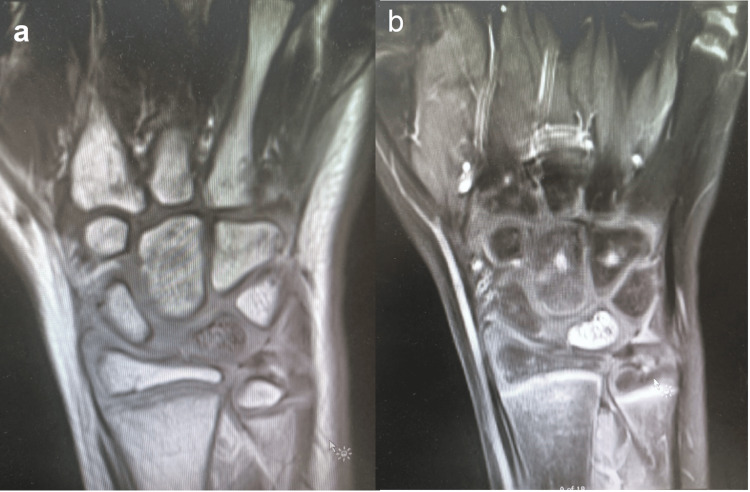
The altered bone marrow signal of lunate bone, which appears hypointense on T1-weighted image (a) and hyperintense on short tau inversion recovery (STIR) (b), represents a bone marrow edema but an intact cortex with no loss of volume.
